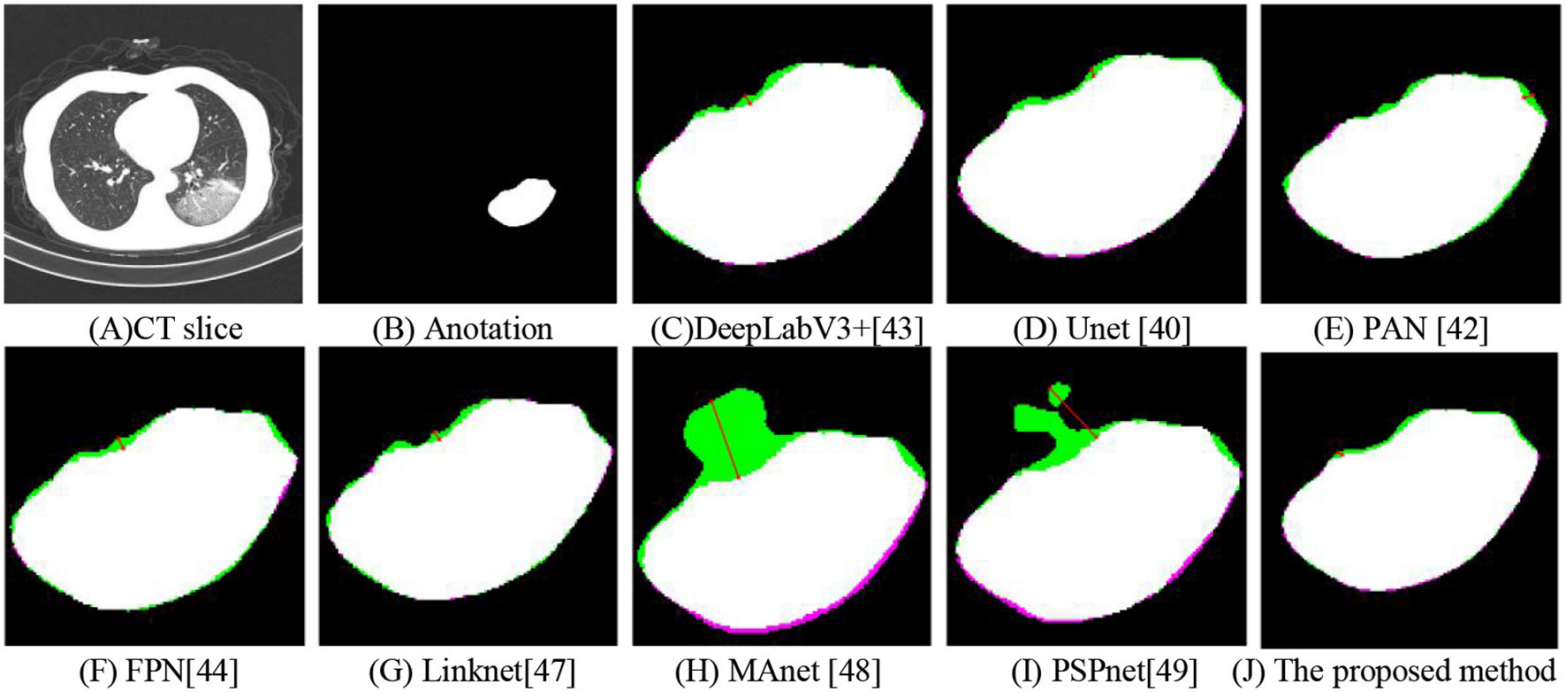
Hausdorff distance with different methods. (A) CT slice. (B) Anotation. (C) DeepLabV3+. (D) Unet. (E) PAN. (F) FPN. (G) Linknet. (H) MAnet. (I) PSPnet. (J) The proposed method.
